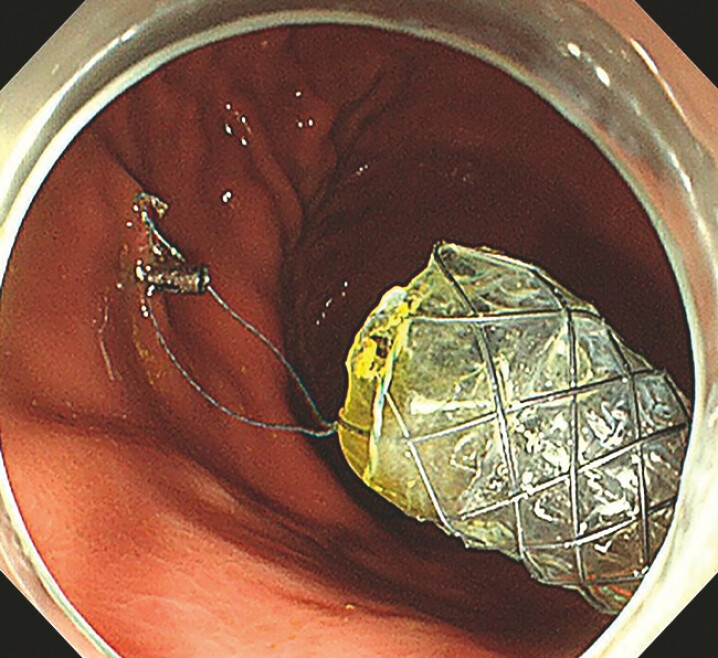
The placement of a fully covered self-expandable metallic stent (FCSEMS) across the endoscopic ultrasound-guided hepaticogastrostomy route was followed by fixation of an endoclip on the opposing gastric wall to prevent stent migration.
